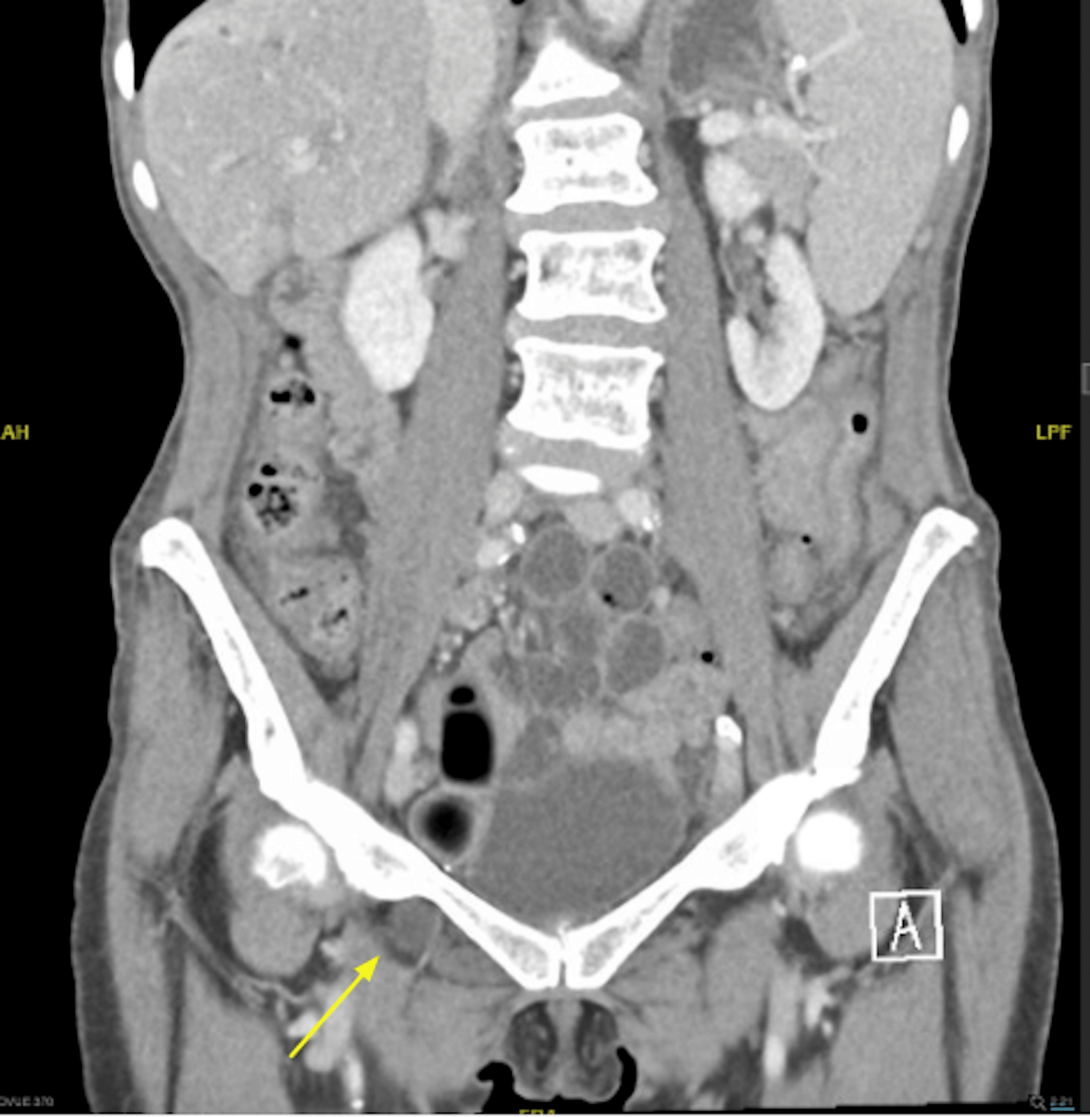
Coronal CT of the abdomen and pelvis demonstrating a portion of the small intestine protruding into the right obturator canal (yellow arrow)
